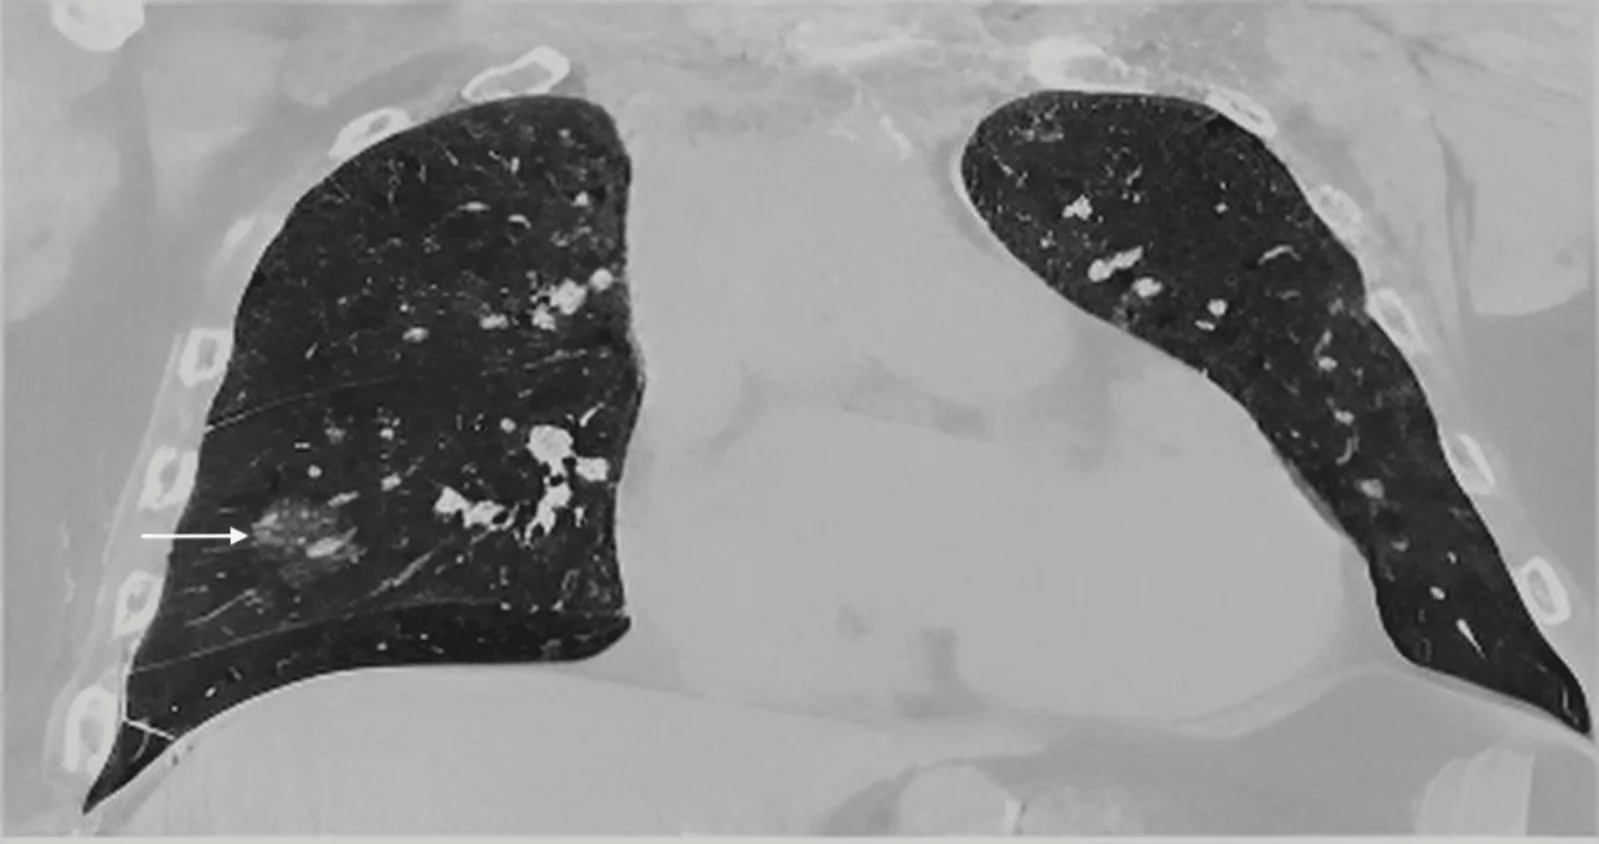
Non-contrast chest CT, coronal view. The arrow indicates a nodular lesion in the right lower lobe.
